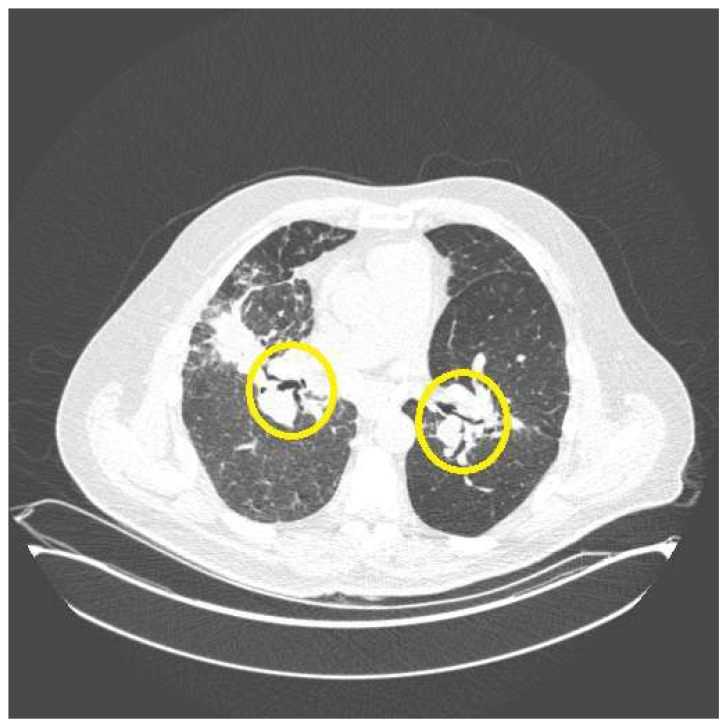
Chest CT scan of a patient with fibrotic pulmonary sarcoidosis. Distortion of airways (yellow circles) is common in this condition and is the result of granulomatous-induced airway fibrosis.
